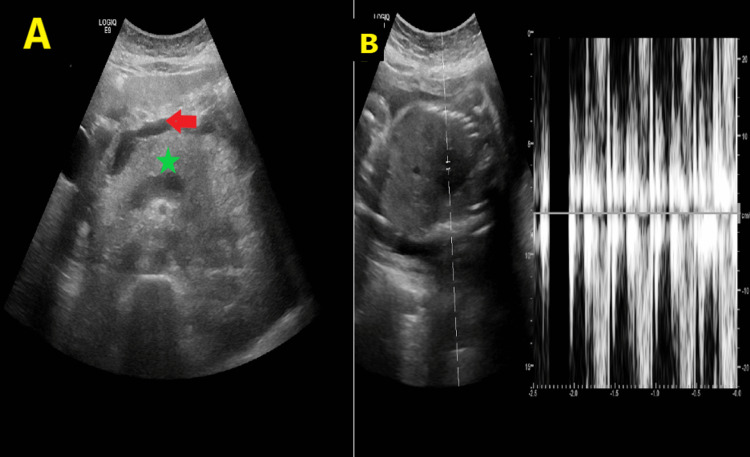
A: Abdominal ultrasound study for the pregnant patient at 33 weeks showing diffusely edematous and swollen pancreas (green star) with loss of its feathery appearance and associated peripancreatic free fluid collection (red arrow), impressive of acute pancreatitis. B: Gravid uterus showing single viable intrauterine fetal pregnancy.
